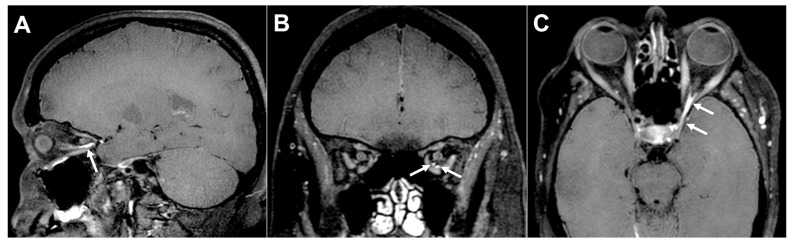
Post-contrast black-blood MRI demonstrating enhancement of the left oculomotor nerve (OMN) consistent with inflammatory neuropathy. (A) Sagittal, (B) coronal, and (C) axial post-contrast black-blood T1-weighted images demonstrate smooth, linear enhancement with only mild thickening of the cavernous segment of the left OMN, extending anteriorly into its orbital division through the superior orbital fissure (arrows). The enhancement is continuous along the expected anatomic course of the nerve, without nodularity or a discrete mass. The adjacent cavernous sinus is not expanded, and there is no evidence of vascular abnormality or compressive lesion. The imaging findings support inflammatory oculomotor neuritis as the underlying cause. The patient had presented with diplopia and ptosis. Image Credit: Dr. Nikolaos-Achilleas Arkoudis and Prof. Georgios Velonakis.
